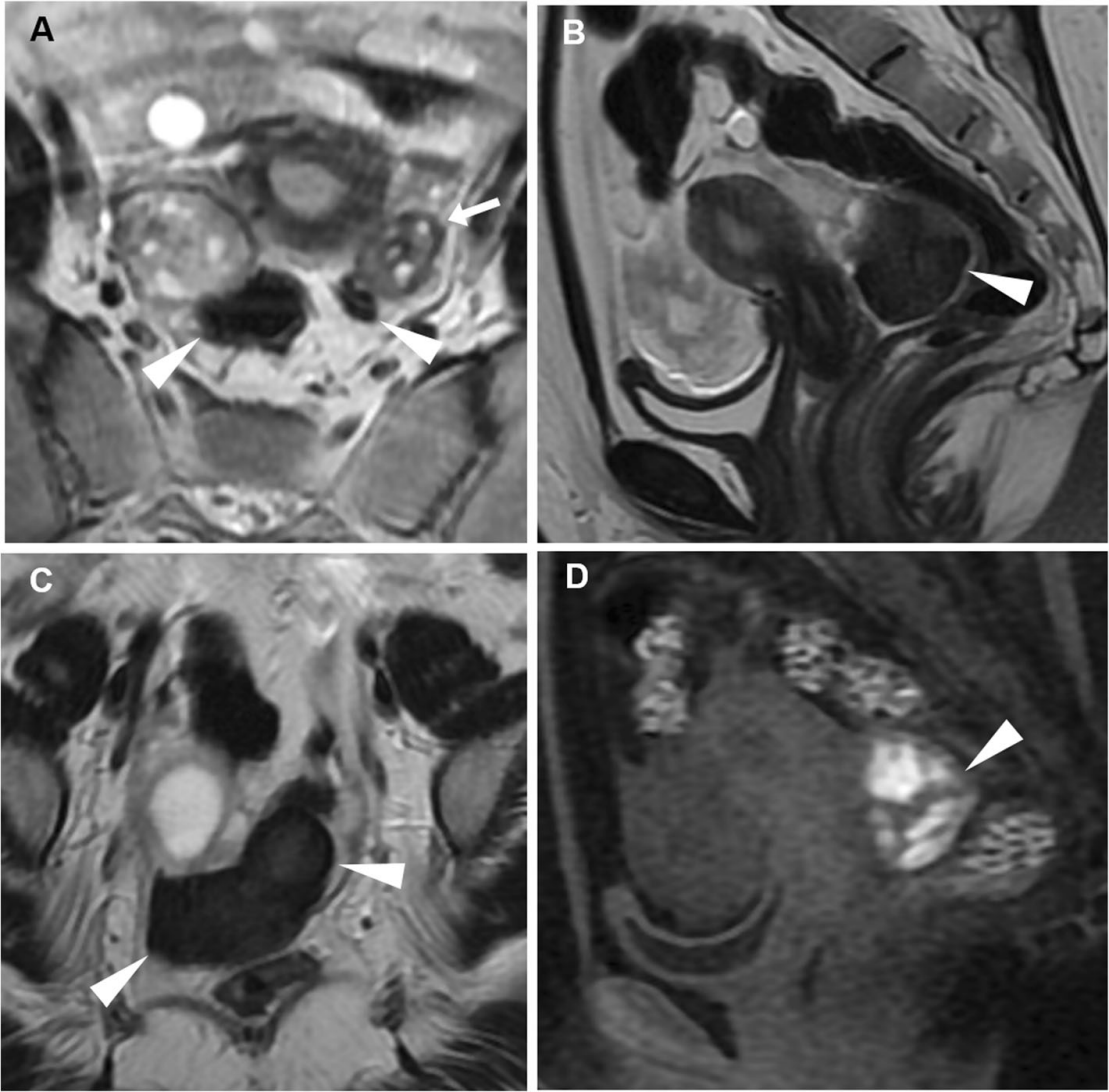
Ruptured corpus luteum in a 20-year-old woman with pelvic pain and fever over the previous 2 days. Oblique-axial (a), sagittal (b) and oblique-coronal (c) T2-weighted and sagittal precontrast fat-suppressed T1-weighted (d) images show a haemorrhagic collection (arrowheads), T2-hypointense and T1-hyperintense, in the pouch of Douglas, contiguous to the left ovary (arrow in a). The latter represents the source of bleeding, although the corpus luteum is no longer recognizable
